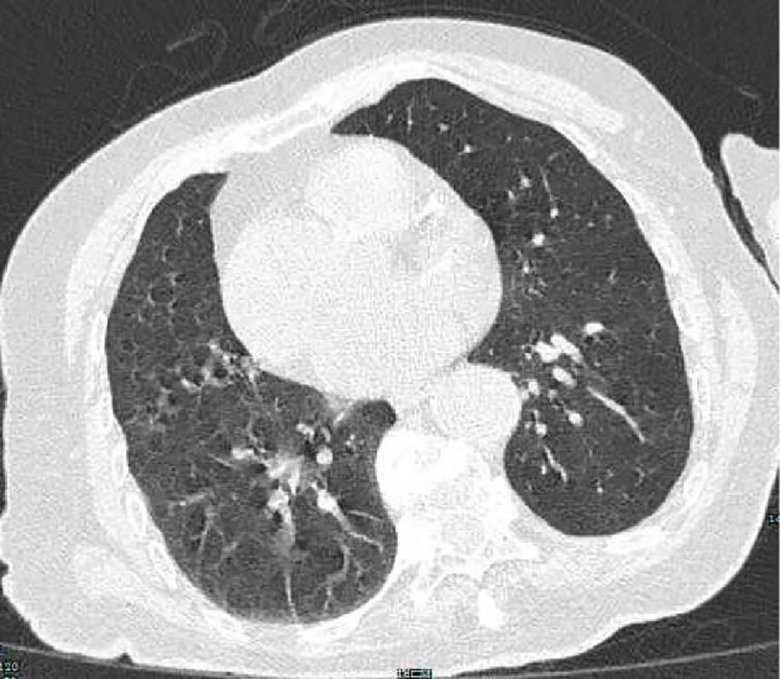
The chest CT scan revealed no tumors or tuberculosis, showing only bronchial lesions.
